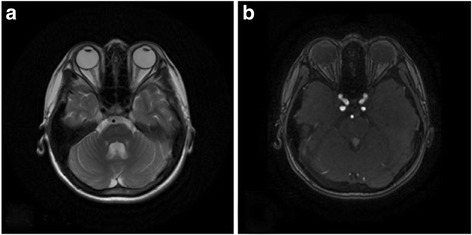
Cranium MRI. MRI shows bilateral optic nerve atrophy on T2WI (a) and T1W+C (b)
